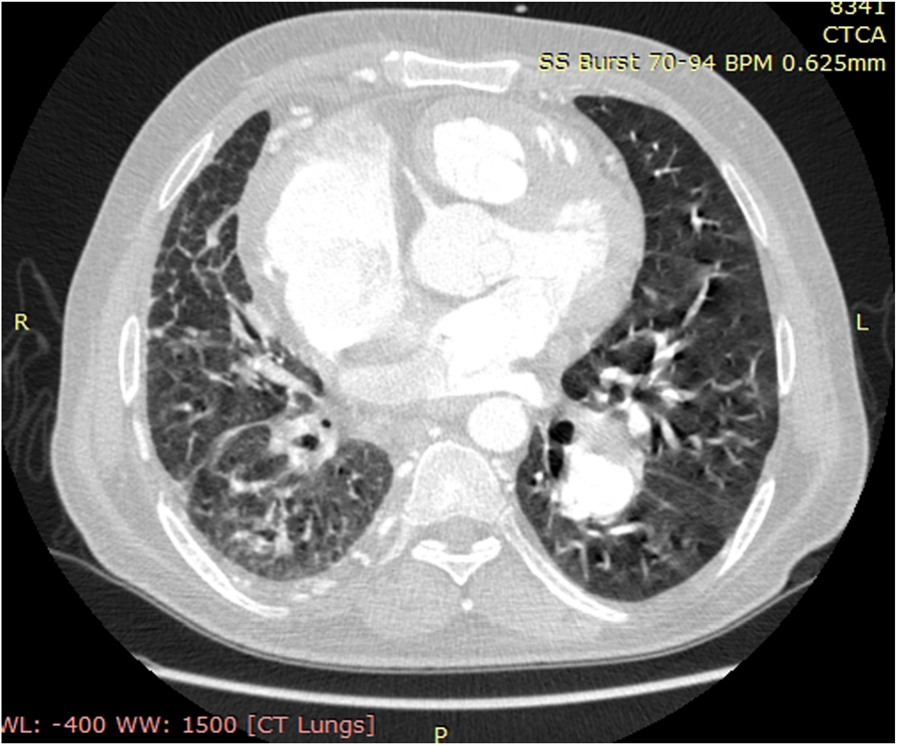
CT chest showing bilateral bronchiectatic changes
